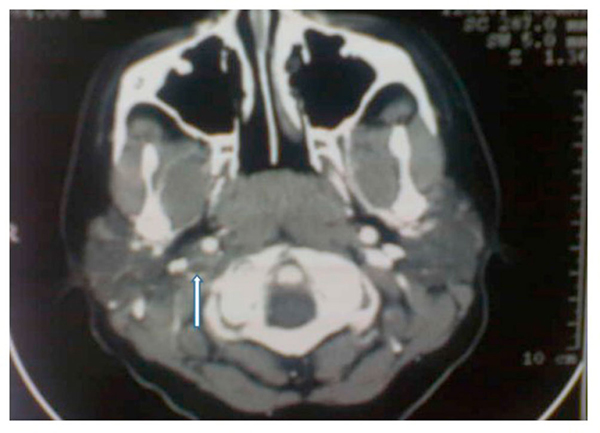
Computed tomography scan of the head: Right internal jugular venous thrombosis (white arrow).
